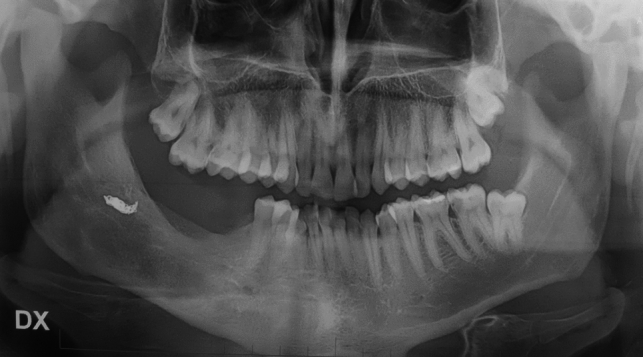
The 1-year post-operatory panoramic radiographic
